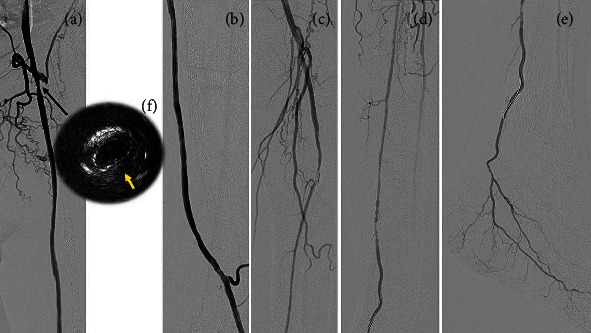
Angiograms immediately after therapy (a–e). (f) IVUS findings immediately after treatment of the proximal anastomosis. Moderate stenosis due to isoechoic plaque (yellow arrow) was noted. IVUS: intravascular ultrasound.
